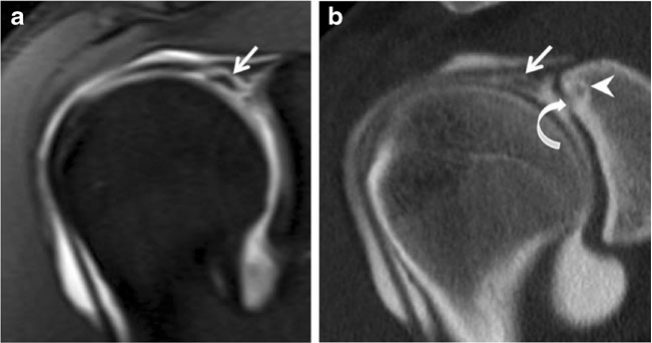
SLAP tear type IV in a 20-year-old goalkeeper after a fall onto fully abducted right shoulder with immediate pain. Coronal (a) fat-suppressed T1-weighted MR arthrography and (b) corresponding coronal CT arthrography image. Bucket handle tear of the labro-bicipital complex extending across the bicipital attachment (*straight arrow*). Note the presence of a small focal cartilage defect of the superior labrum only detected on the CT arthrography image (*curved arrow*) with adjacent subchondral cyst (*arrowhead*) suggesting a chronic chondropathy
